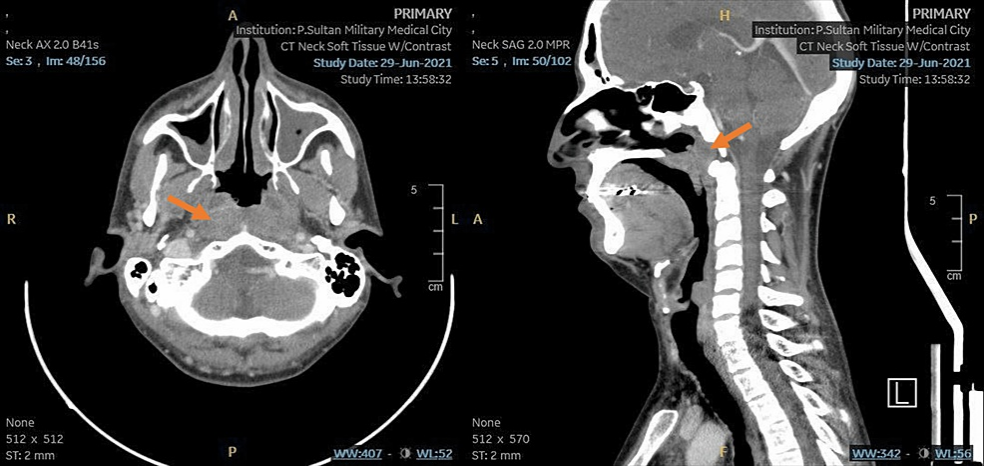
Contrast-enhanced CT of the head and neck, showing a relatively large ill-defined infiltrative heterogeneously enhancing soft tissue mass that is noted in the nasopharynx (orange arrow). It obliterates the fossae of Rosenmuller and pharyngeal openings of both Eustachian tubes with a marked widening of the posterosuperior nasopharyngeal wall. CT, computed tomography
